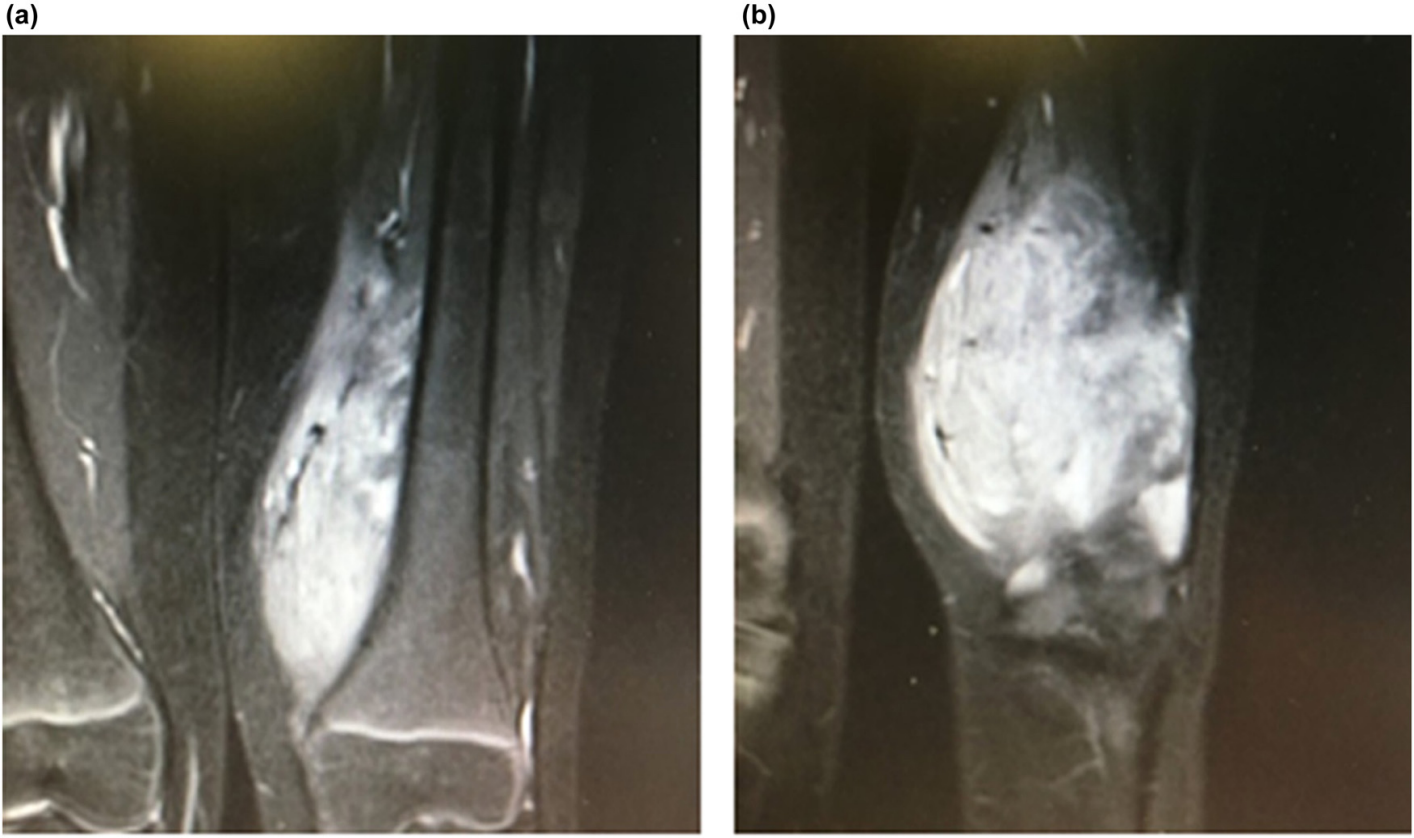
Preoperative magnetic resonance imaging. (a) Focal mass-like FAVA, which produced progressive thigh pain over the last year; (b) the sagittal image shows maximal mass hyperintensity in T2-weighted images.
